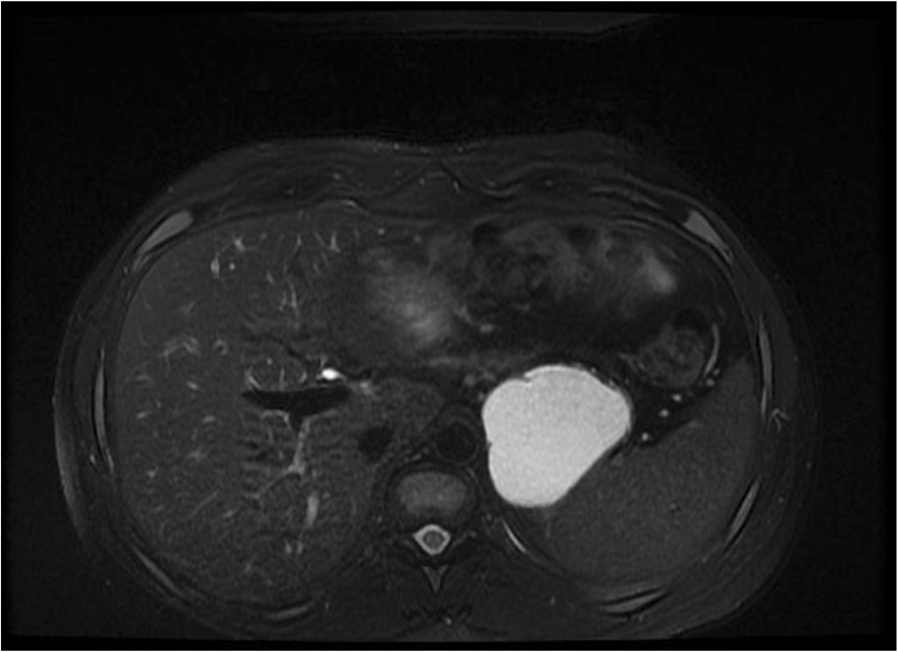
T2-weighted fat-saturated magnetic resonance image shows a high-signal-intensity cystic mass between stomach and spleen
